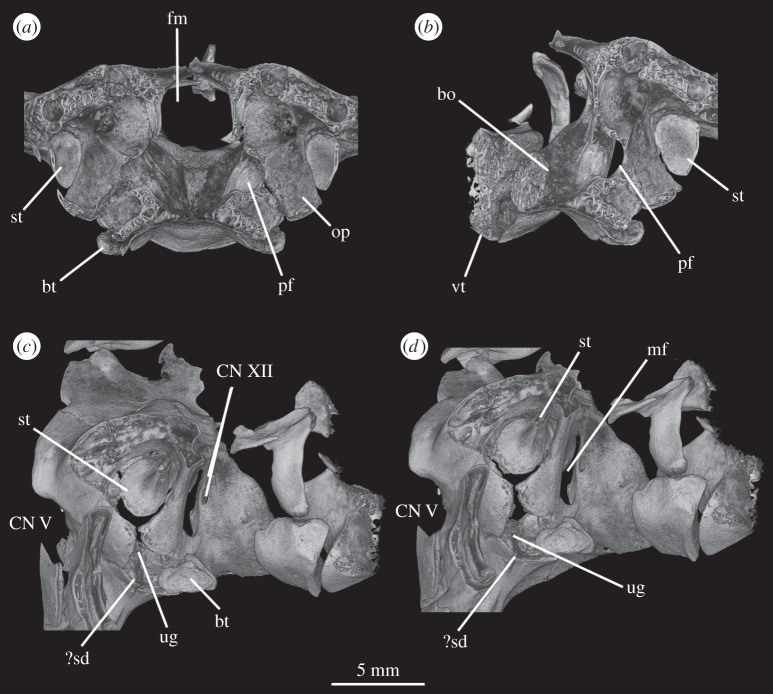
CT reconstructions of braincase of *Sphenodon punctatus* (ROM R9298): (*a*) anterior view in transverse cross section, (*b*) anteromedial view in cross section, (*c*) left lateral view and (*d*) ventroposterolateral view. For abbreviations, see table 1.
